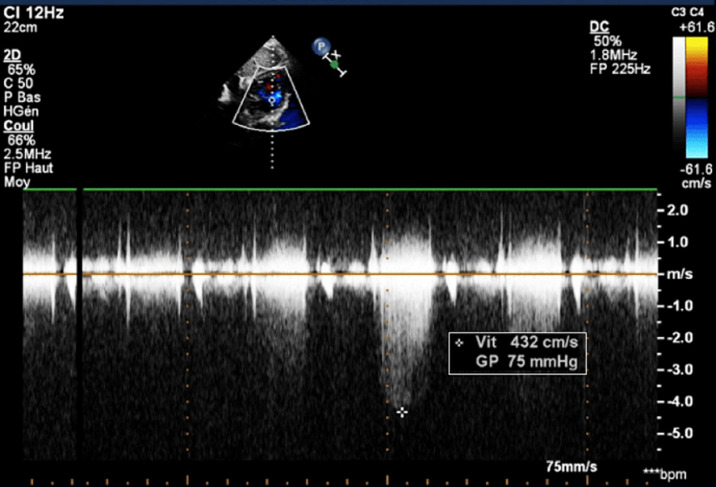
continuous wave Doppler across right ventricular outflow tract in parasternal short-axis view showing a gradient of 75 mmHg
